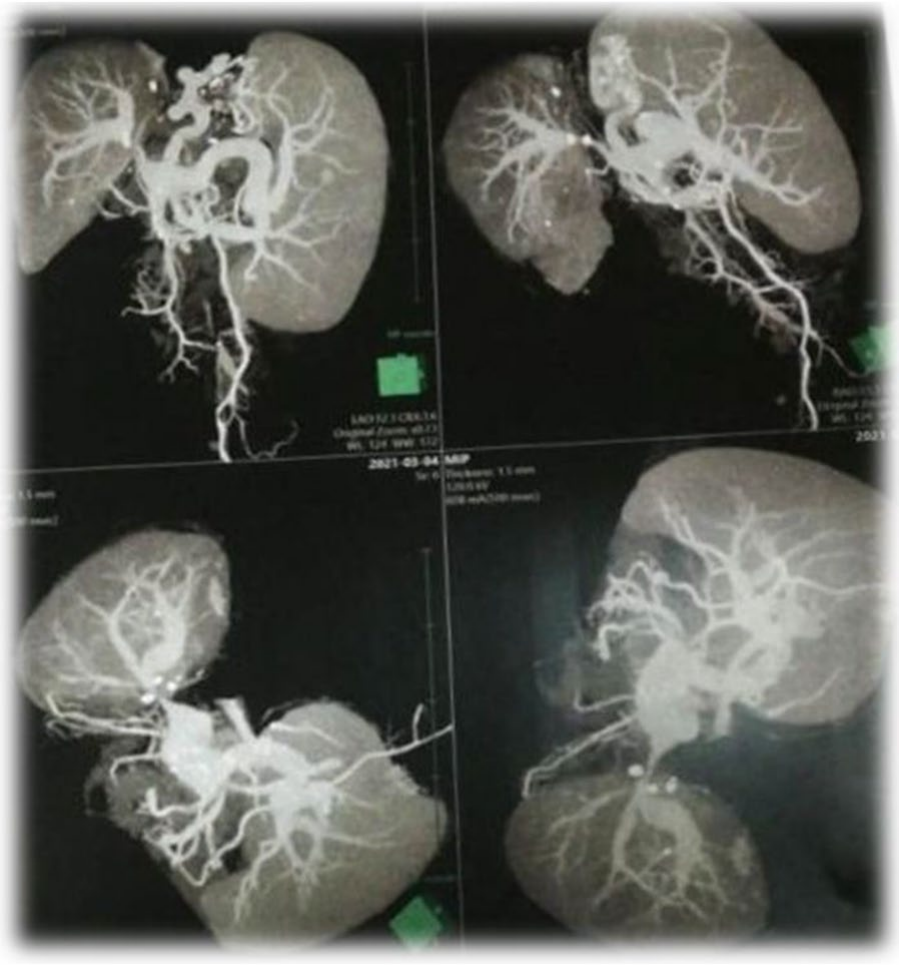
CT angiography reconstruction images showed significant luminal stenosis at site of anastomosis of the portal vein
